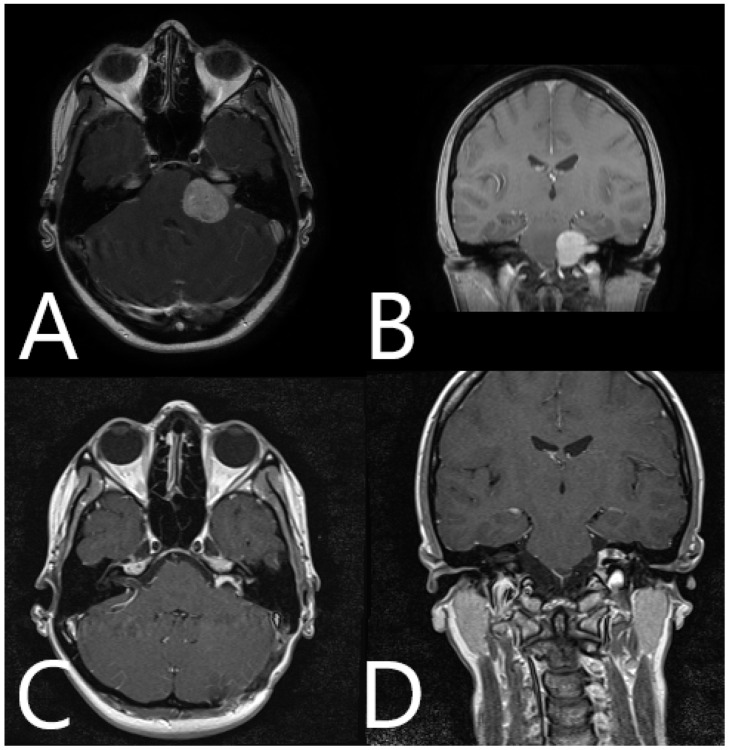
Patient No. 38. Preoperative (A) axial and (B) coronar T1-weighted post-contrast MRI with postoperative (C) axial and (D) coronar T1-weighted post-contrast MRI, depicting subtotal resection of the tumor and slight contrast enhancement along the course of the facial nerve.
